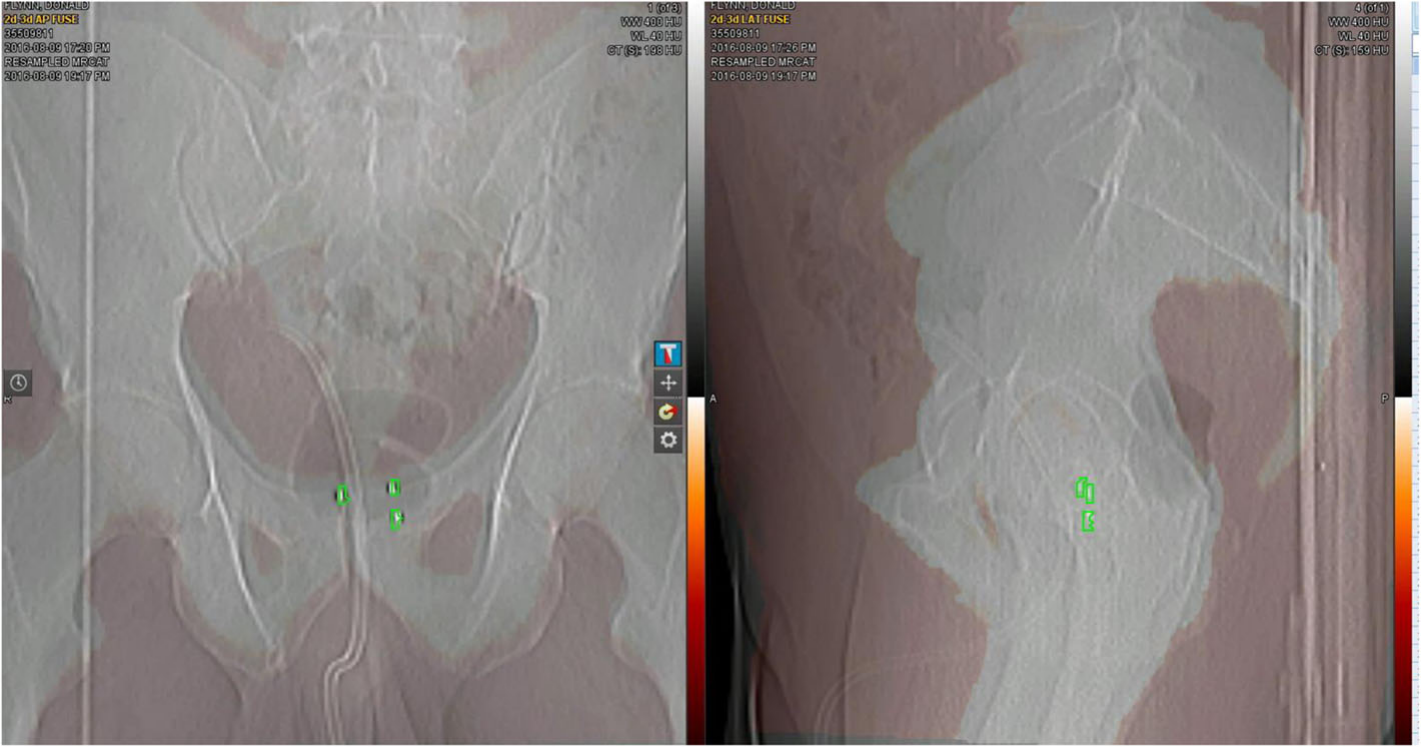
3D-2D registration between the MRCAT syn-CT and CT orthogonal scouts
